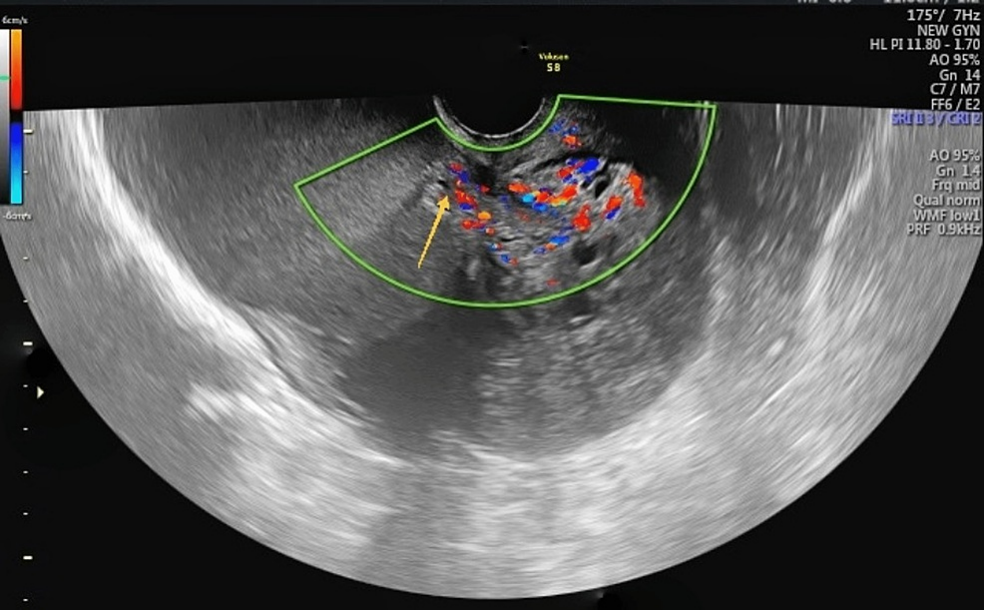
Ultrasound findings of exophytic adenomyoma TVUS image showing a focal ill-defined cystic heterogenous lesion (arrow) in the posterior uterine wall, penetrating the serosa with increased vascularity (Colour score: 3)
